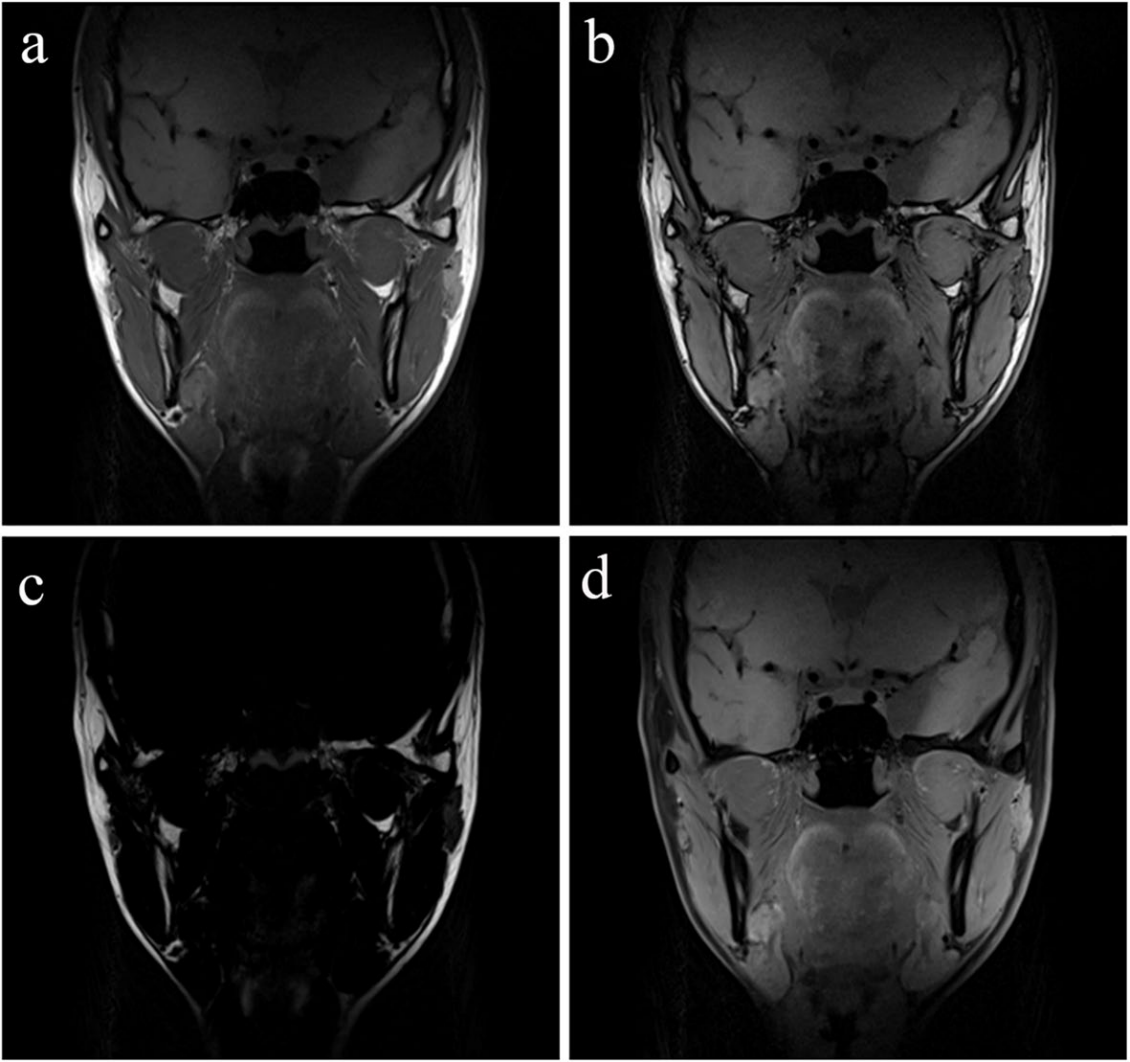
Four phases of images obtained by coronal T1-weighted Dixon sequence. (A) In-phase image. (B) Opposed-phase image. (C) Fat image. (D) Water image
